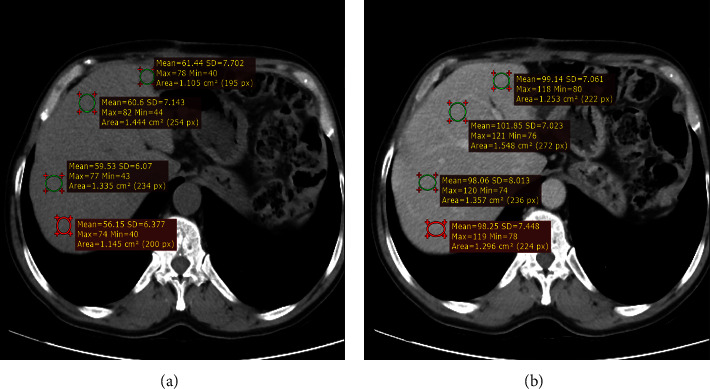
Axial precontrast (a) and postcontrast portal venous phase (b); axial CT scans at the same level taken in TASH, 2022 G.C, show mean hepatic attenuation at the four different locations of measurement.
